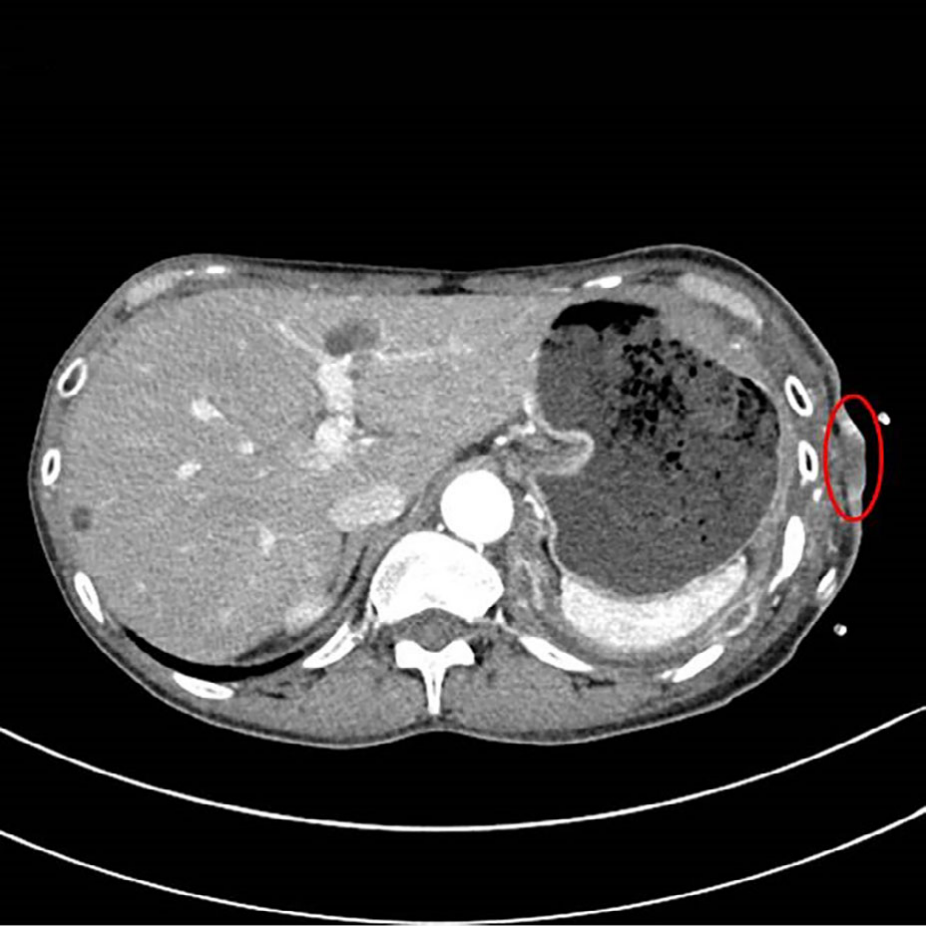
Computed tomography of the chest showing the presence of a soft tissue mass protruding out of the subcutaneous tissue through the skin on the left (highlighted with red oval).
